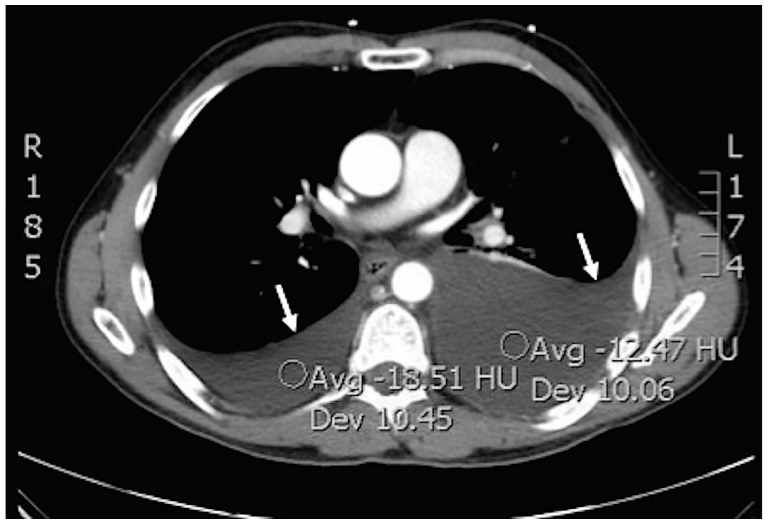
Computed tomography of the chest with contrast showing bilateral pleural effusions more remarkable on the left side (white arrows).
